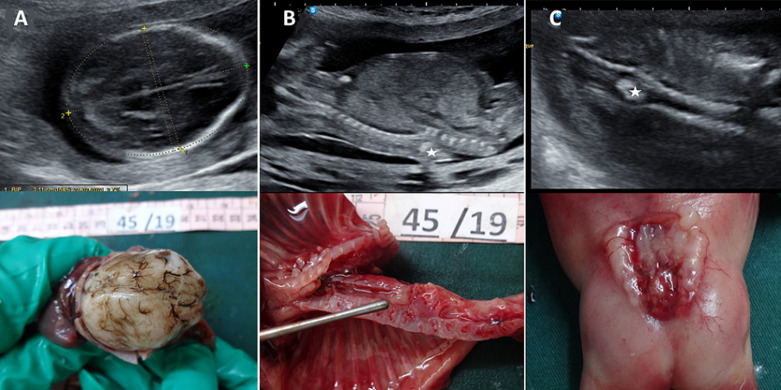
(A, B, C) prenatal sonography findings and fetopathology examination
